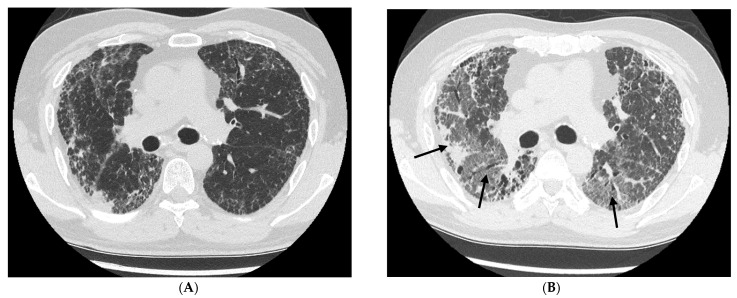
(A) HRCT of patient 4 with fNSIP pattern before AE-ILD. (B) HRCT of patient 4 with fNSIP pattern during AE-ILD. Arrows indicate newly developed consolidations and GGOs superimposed on a fNSIP pattern.
